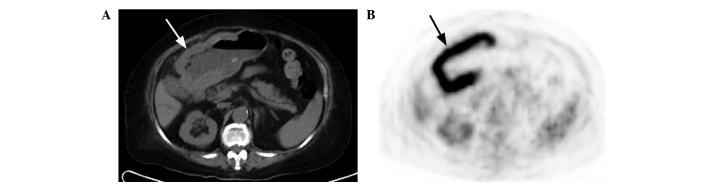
A 81-year-old female with gastric poorly-differentiated adenocarcinoma. (A) In the axial computed tomography image, a diffuse thickened gastric wall can be observed in the body and the antrum of the stomach. (B) In the axial positron emission tomography image, diffuse increased ^18^F-fluorodeoxyglucose accumulation (maximal standard uptake value, 18.5) can be confirmed in the corresponding gastric regions.
